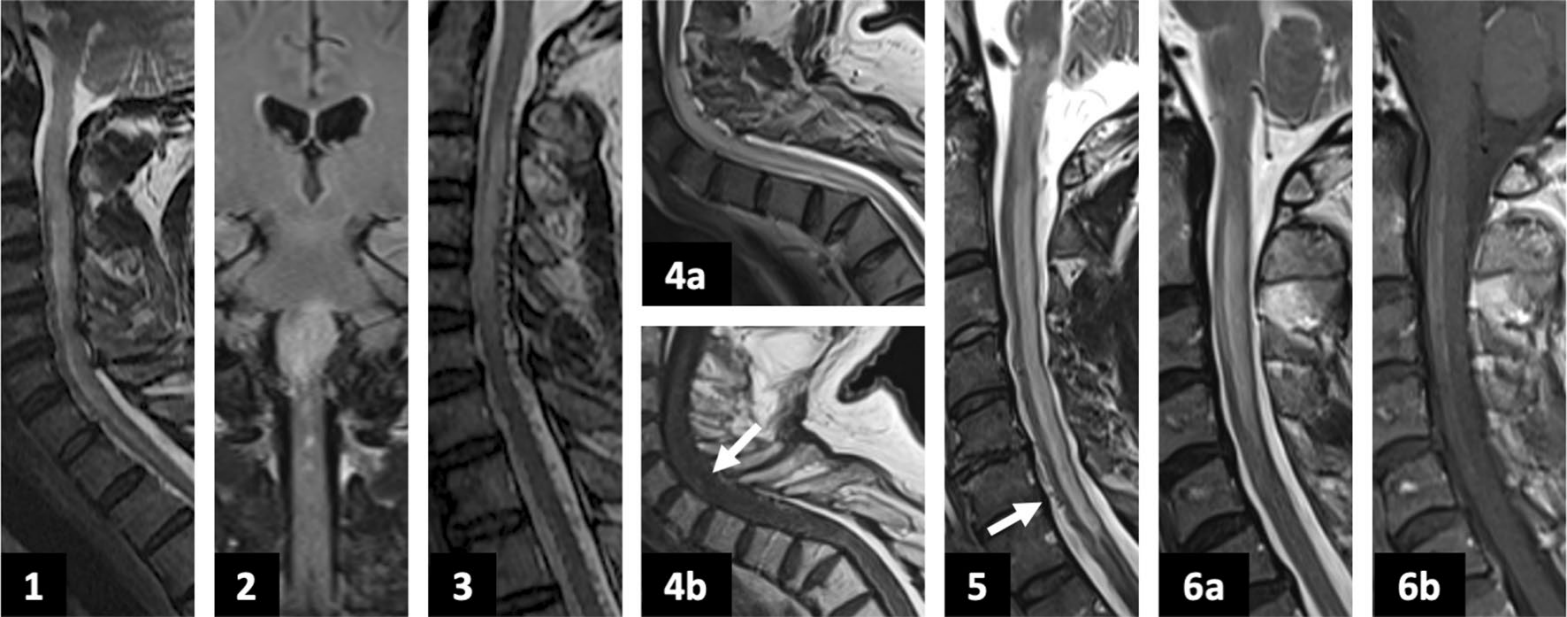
MRI images at presentation in each of the cases. (1) Case 1: sagittal T2-weighted image showing cervical cord swelling and signal abnormality from the cervicomedullary junction to C5. (2) Case 2: coronal T2-FLAIR image showing signal abnormality in the pons and medulla. (3) Case 3: sagittal T2-weighted image showing intramedullary signal abnormality at C2–C7 with cord expansion. Numerous flow voids are visible on the dorsal surface of the cord, indicating dilated perimedullary vessels. (4a) Case 4: sagittal T2-weighted image showing intramedullary signal abnormality at C3–T3 with cord expansion. (4b) Case 4: sagittal gadolinium-enhanced T1-weighted sequence showing subtle pathological gadolinium enhancement in the anterior cord (white arrow). (5) Case 5: sagittal T2-weighted image showing intramedullary signal abnormality from the pontomedullary junction to T1. There are some subtle flow voids on the cord surface (white arrow), but these were initially felt to relate to the patient’s history of an intramedullary cavernoma at C7/T1 surgically treated 9 years prior. (6a) Case 6: sagittal T2-weighted image showing intramedullary signal abnormality from C1–C5 with cord expansion. (6b) Case 6: sagittal gadolinium-enhanced T1-weighted sequence showing pathological gadolinium enhancement in the anterior cervical cord at C1–C5
